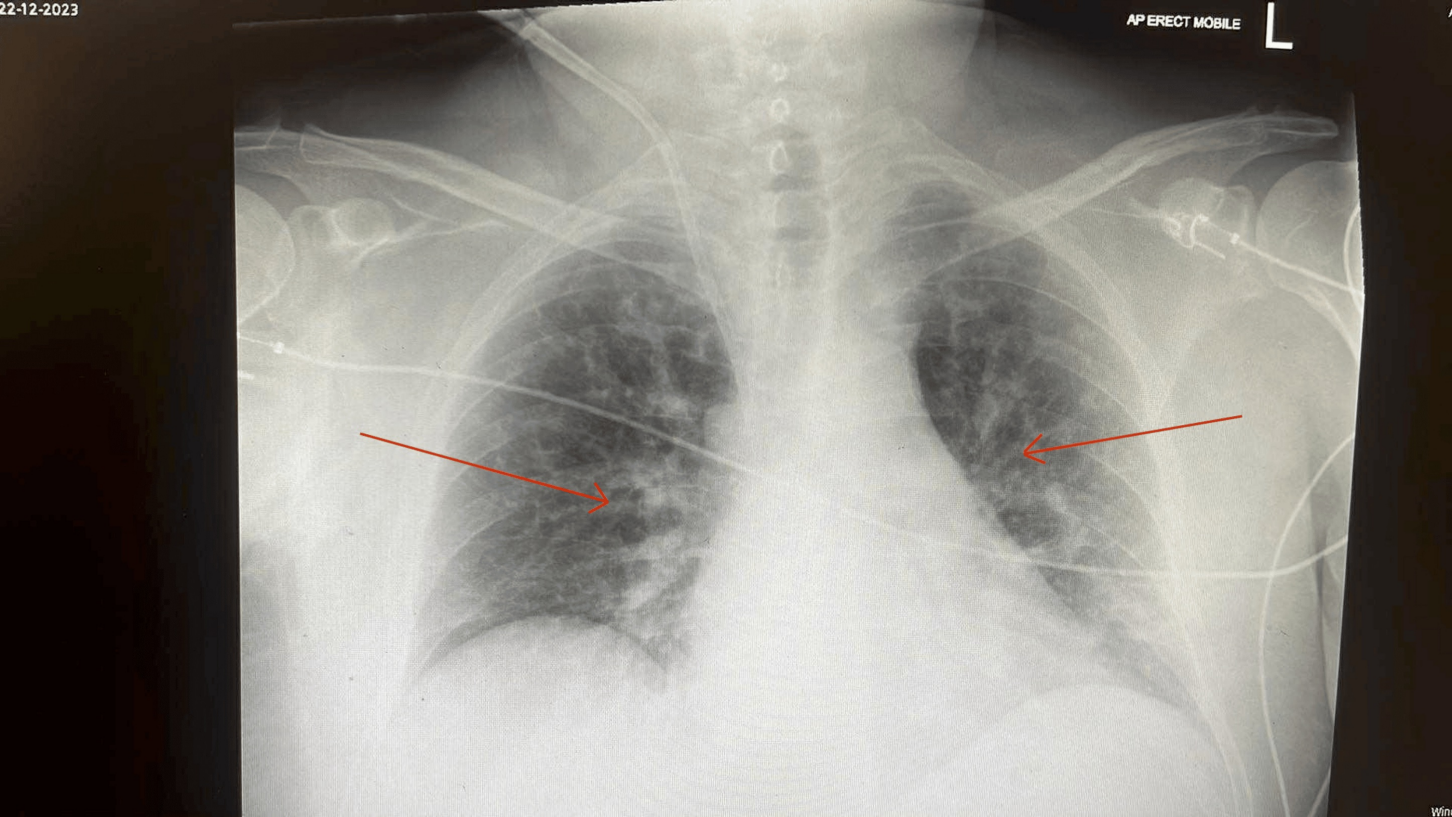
Anteroposterior Chest X-Ray Arrows highlighting increased lung markings suggestive of interstitial oedema
